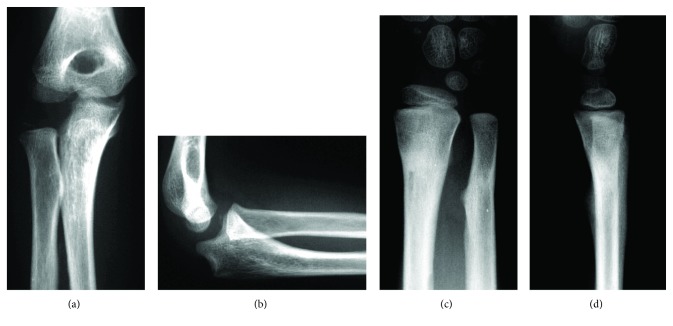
X-rays 3 months after surgery. (a) Front view of the elbow joint. (b) Lateral view of the elbow joint. (c) Front view of the wrist joint. (d) Lateral view of the wrist joint.
